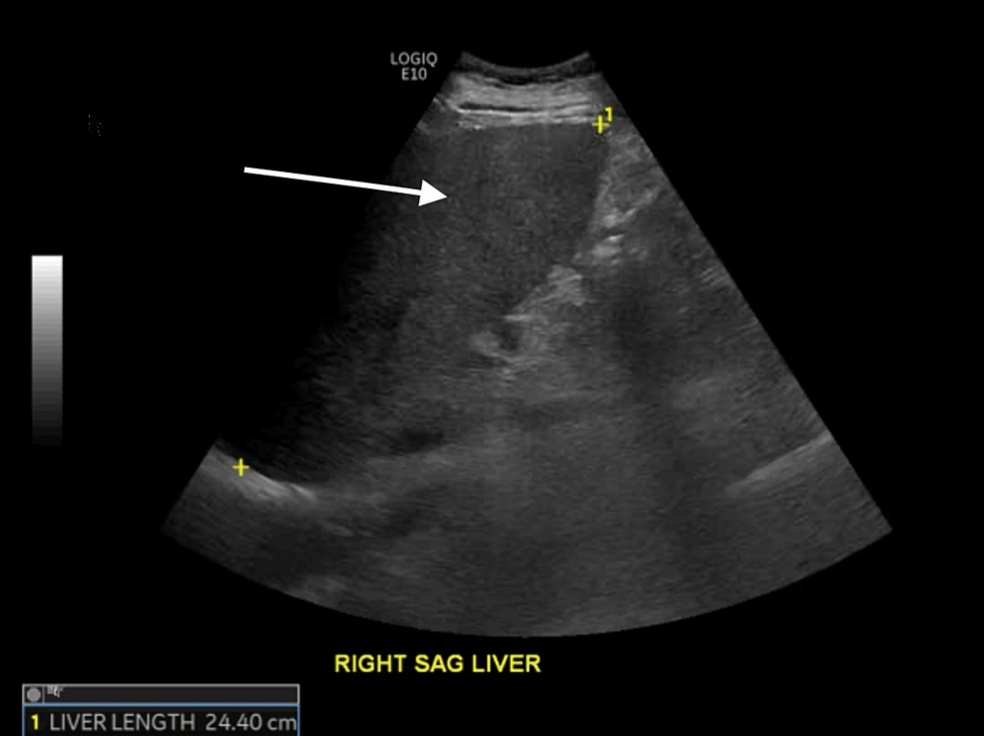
Abdominal ultrasound Abdominal ultrasound showing reduced hepatic echogenicity with hepatic enlargement suggestive of hepatic fatty infiltration (arrow).
